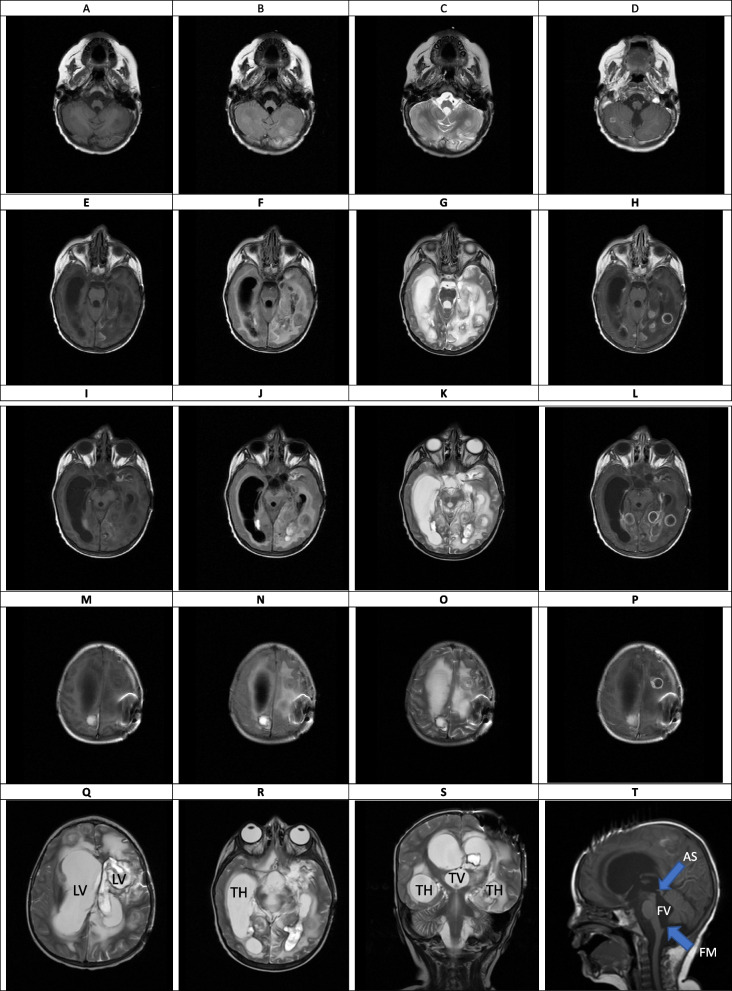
(the Brain MRI Without and with contrast, day 21). A-T Multiple varying size ring-like enhancing lesions within the different parts of the white matter of both cerebral and cerebellar hemispheres (some of them representing mentioned hypodense lesion within the previous CT SCAN) suggestive of multiple abscess formation. Sign of significant enhancement of ependymal layer of trigone, occipital and temporal horns of left and lesser degree right lateral ventricles is seen as suggestive of ventriculitis. Sign of marked dilatation of lateral ventricles (including temporal horns) as well as third and fourth ventricles and also aqueduct of *Sylvius* and foramen *Magendie*, all suggestive of communicating type of hydrocephalus (which is most likely secondary to the previous intraventricular hemorrhage and also ventriculitis, obstructing pores of subarachnoid spaces). LV: lateral ventricle, TH: temporal horn of lateral ventricle, TV: third ventricle, FV: fourth ventricle, AS: aqueduct of *Sylvius*, FM: foramen *Magendie*
